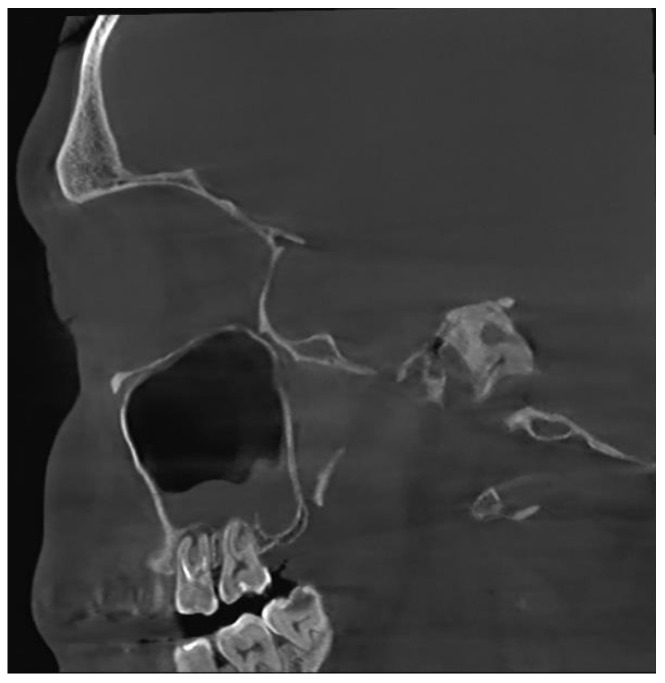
CBCT image of odontogenic sinusitis caused by iatrogenic harm via failed endodontic treatment: upper first and second molar periodontitis, chronic apical periodontitis (sagittal view).
